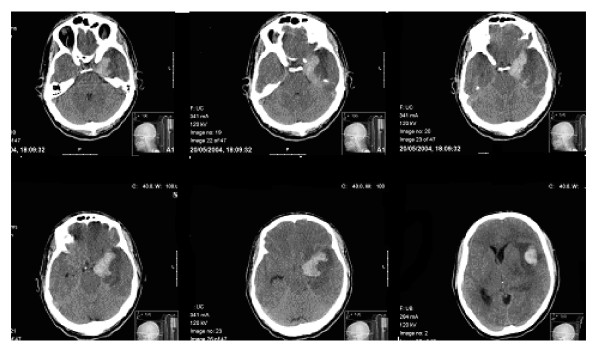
Preoperative computerized tomography(CT) scan. The scan shows intracranial hemorrhage over the left temporal lobe and right frontal subdural hemorrhage compressing the central line of the brain contralaterally.
